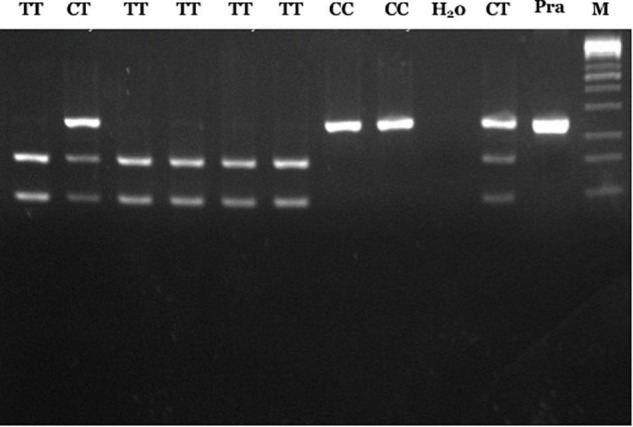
PCR-RFLP results of *RET* rs2435357 variant. Lane 1, 3–6: TT genotype (156 and 90 bp); lane 2, 10: CT genotype (246, 156, and 90 bp), lane 7–8: CC genotype (246 bp), lane 9: H_2_0, lane 11: pra-digested PCR, and lane M: 100 bp DNA marker.
